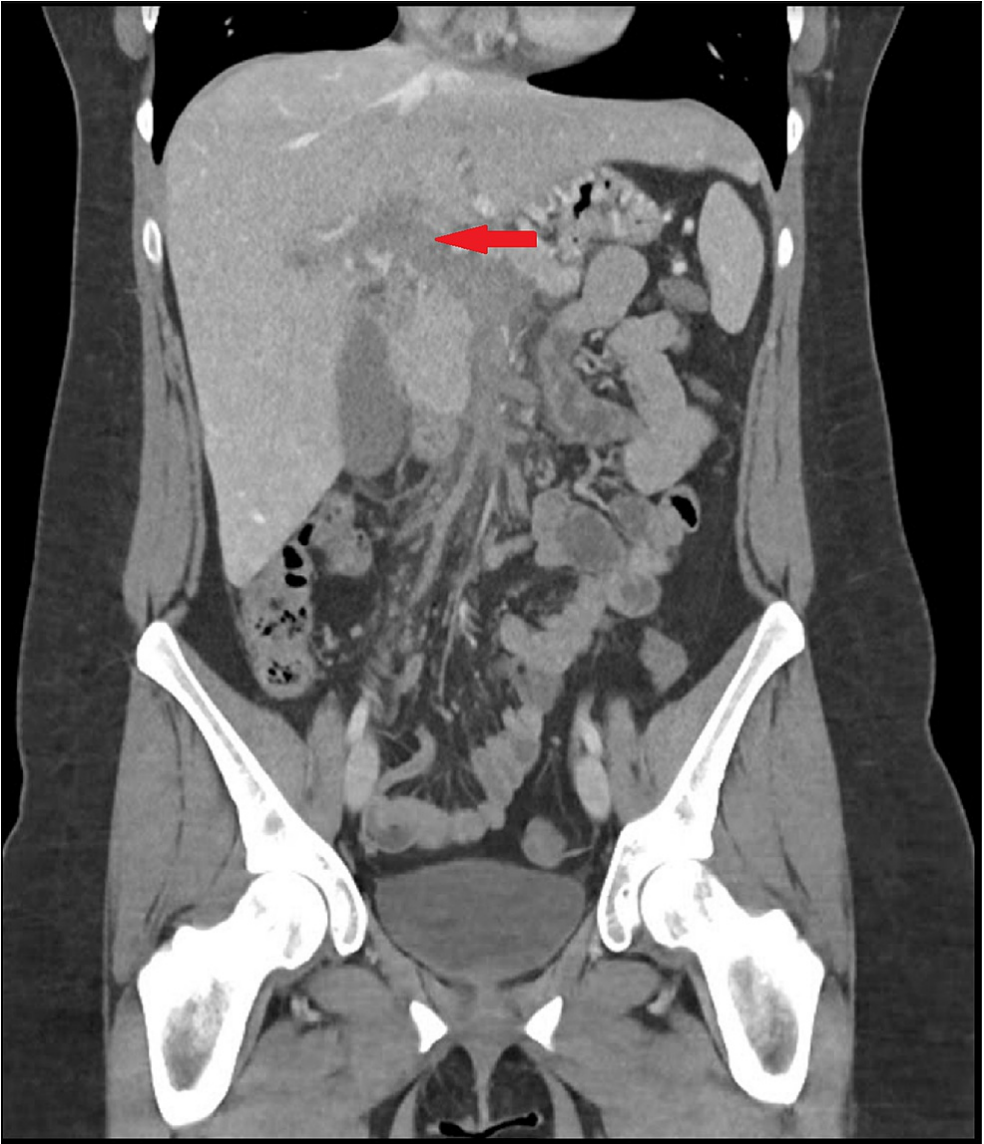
Initial CT imaging Coronal section of initial CT on diagnosis showing portal vein thrombosis
